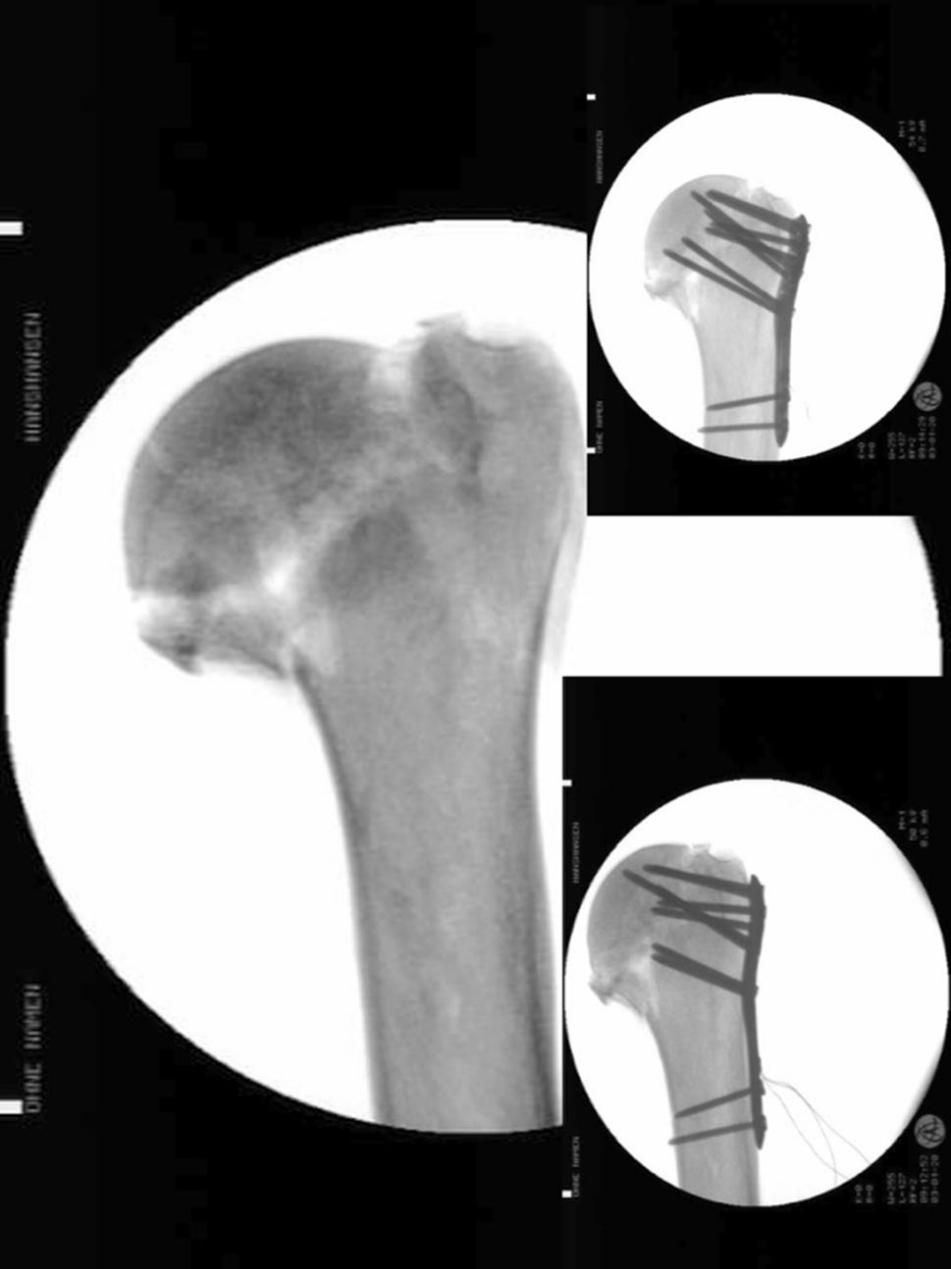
Radiographic scan (a.p.—view) of 3-part humeral fracture before and after locking plate with short and long calcar screws
